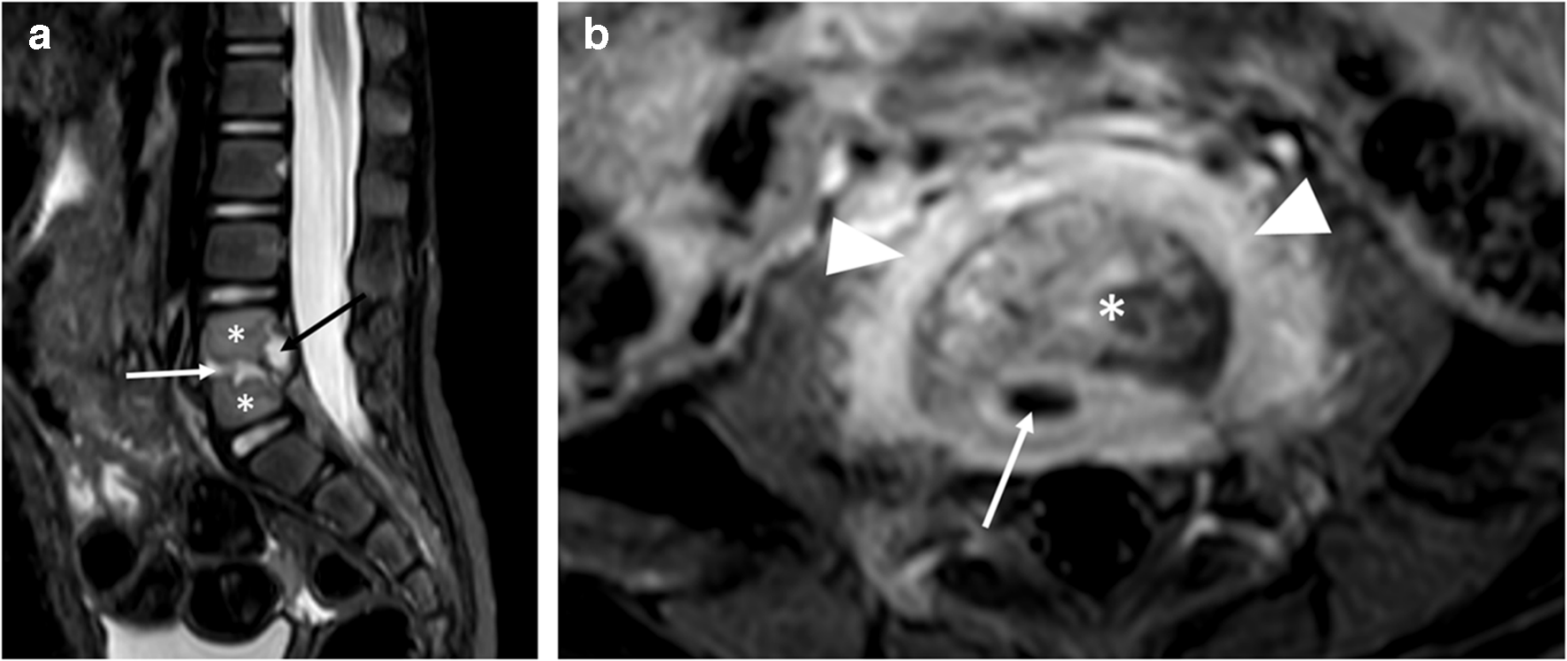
Magnetic resonance images of the lumbar spine in a 21-month-old girl with spondylodiscitis, performed 2 days after the ultrasound (Fig 1). a Sagittal short tau inversion recovery image shows an irregular fourth and fifth lumbar intervertebral disc, with ill-defined endplates (*white arrow*), increased vertebral body signal (*asterisks*), and a small epidural fluid collection (*black arrow*). b Axial contrast-enhanced T1-weighted image with fat saturation at the level of the fourth and fifth lumbar disc demonstrates enhancing paravertebral soft tissue (*arrowheads*), and a small epidural fluid collection (*arrow*). The disc has a heterogeneous signal (*asterisk*). This image corresponds to the sonographic view in Fig. 1a
